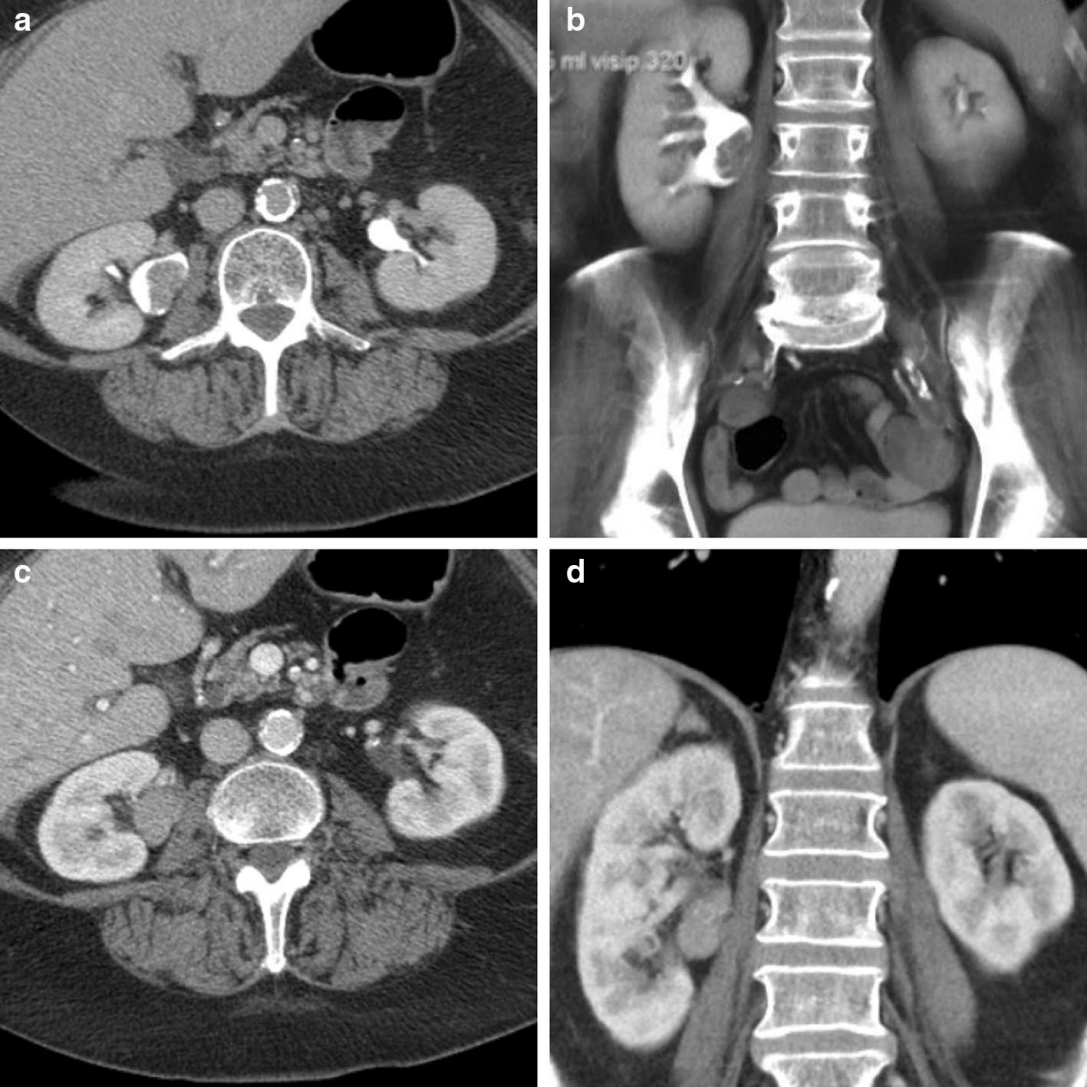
Mutiphase CT urography of a tumor in the right renal pelvis. In the excretion phase (a, b), a filling defect is clearly visible in both the axial and the coronal plane, although this defect may have been caused by a blood clot. In the corticomedullary phase (c, d), the lesion is contrast enhanced, proving that there is tumor growth
